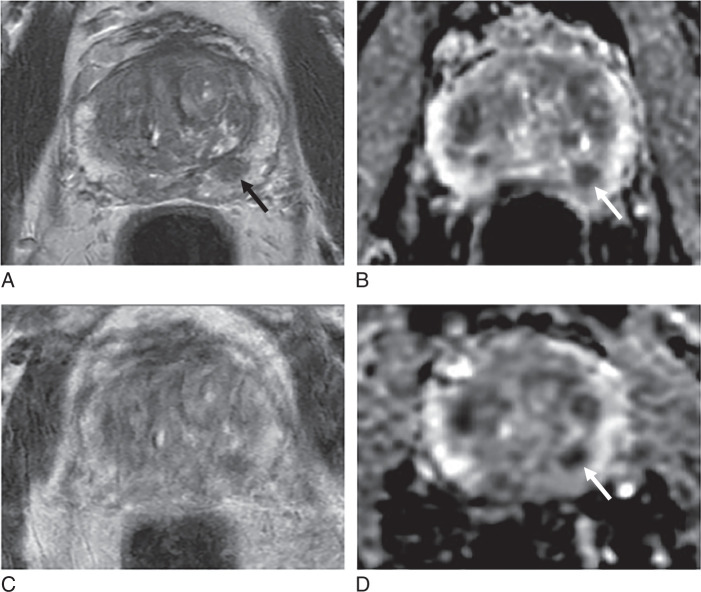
Example of nondiagnostic prone T2w images. Seventy-seven-year-old patient, PSA 4.67 ng/mL. A and B, Supine imaging shows a 9 mm area of focal low T2 signal in the left mid PZ (arrow, A) with restricted diffusion on ADC maps (arrow, B), PI-RADS 4. C and D, Prone T2 is nondiagnostic, PI-QUAL 0/4 (C), ADC map again shows a 9 mm area of marked restricted diffusion (arrow in D). Score is determined by the dominant PZ sequence of DWI and is overall PI-RADS 4 on prone and supine imaging. Targeted biopsy shows Gleason 3+4=7 in 1/3 cores with 6 mm max tumor length.
